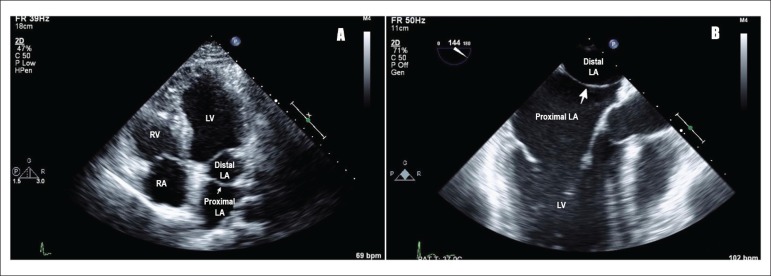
A) Transthoracic echocardiogram showing cor triatriatum: proximal and distal
